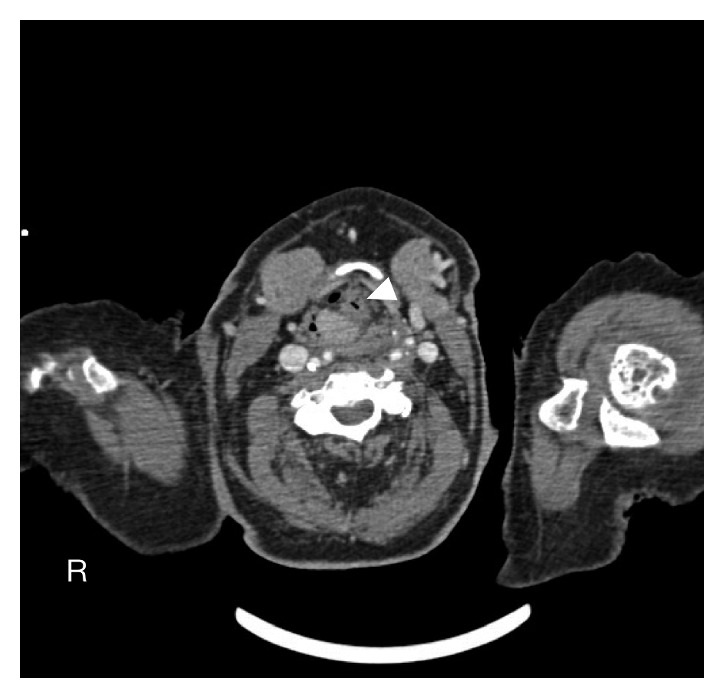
Contrast-enhanced computed tomography scan of the neck shows a large (18 × 22 × 12 mm) solid hypopharyngeal-laryngeal mass with well-defined margins, originating from the right pyriform sinus with partial contralateral extension (white arrow).
